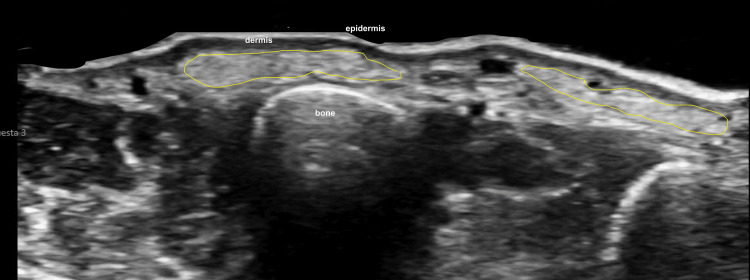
Sonographic appearance of CaHA filler in the dorsal hand (transverse view) Yellow boundary lines outline well-defined hyperechoic bands with a characteristic “cloud-like” configuration corresponding to CaHA deposits (Radiesse®; Merz Aesthetics, Raleigh, NC, USA). Abbreviations: CaHA, calcium hydroxylapatite.
